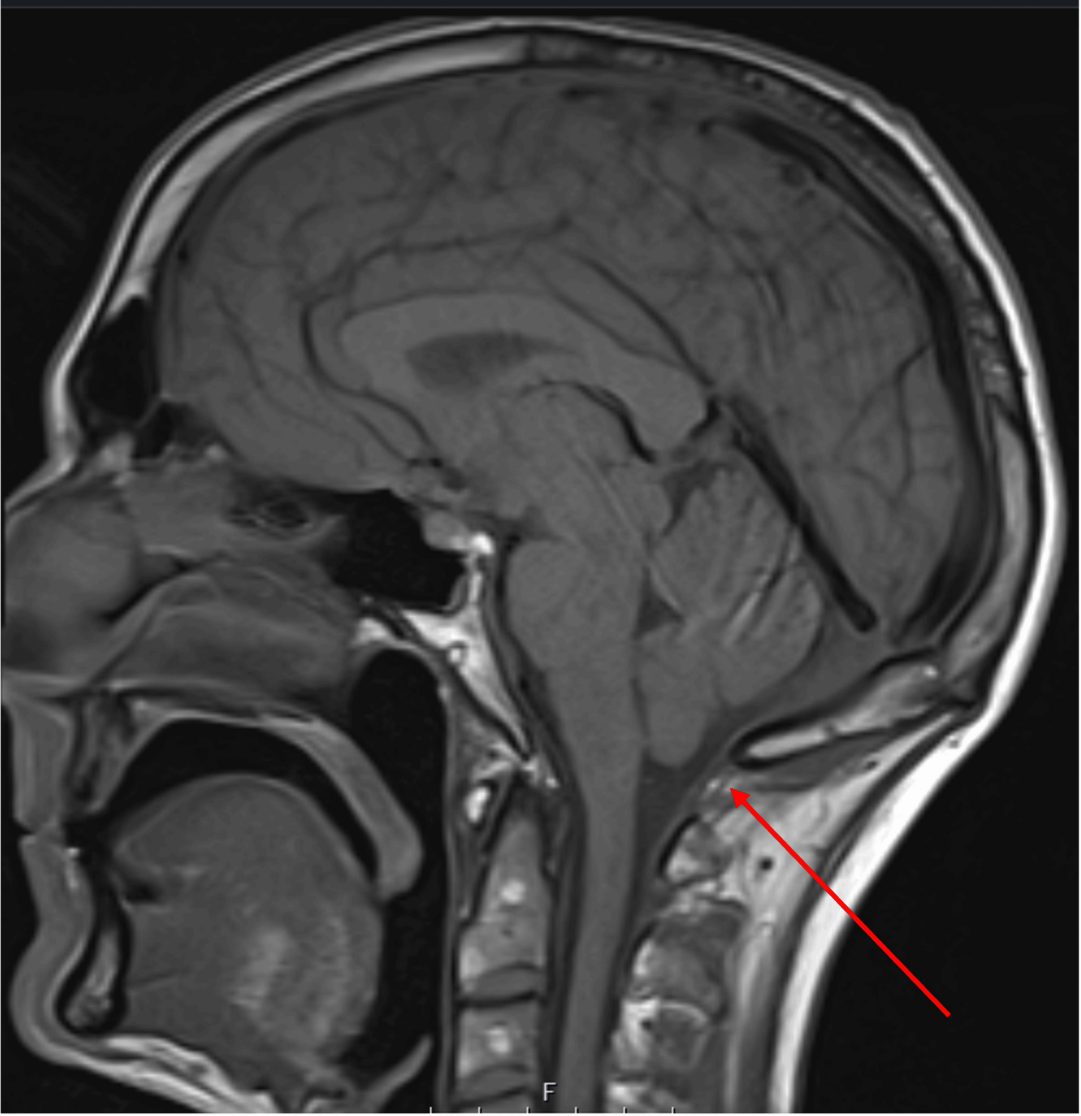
MRI of the head showing slight herniation of the cerebellar tonsils
